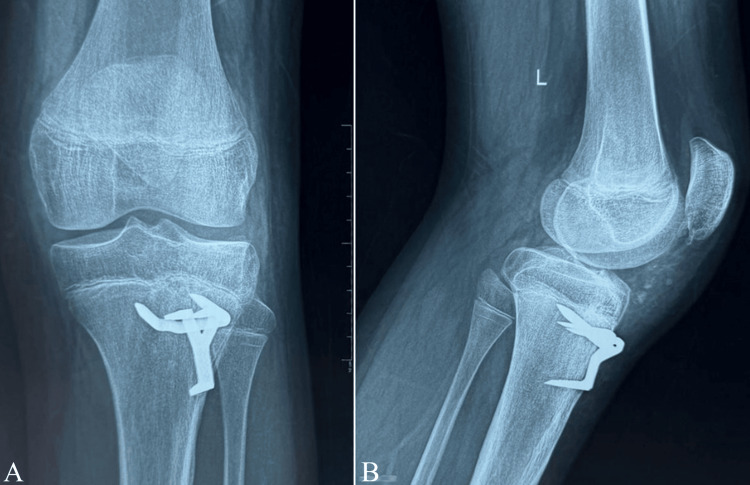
Postoperative radiographs of the left knee six months after surgical fixation and subsequent knee immobilization, in anteroposterior (A) and lateral (B) views
